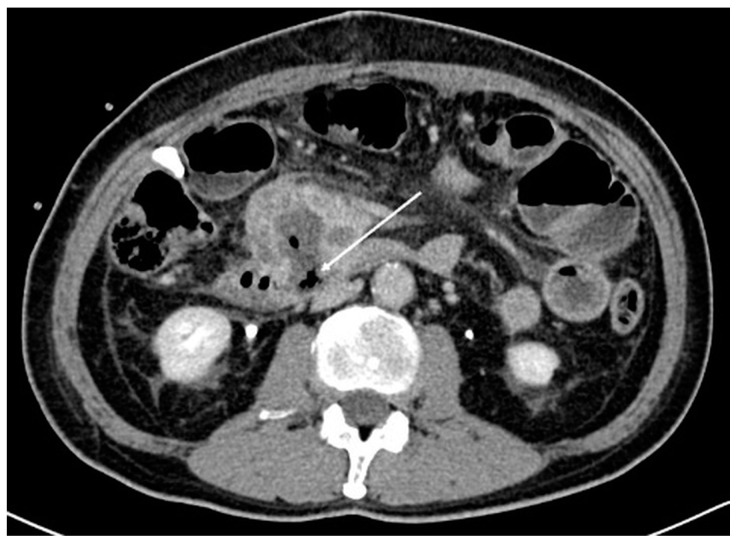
Axial computed tomography image post open irreversible electroporation. At the location of the tumor invasion of the duodenum, there is a defect in the wall with gas seen (arrow). This likely represents perforation of the duodenum due to breakdown of the ablated tumor.
